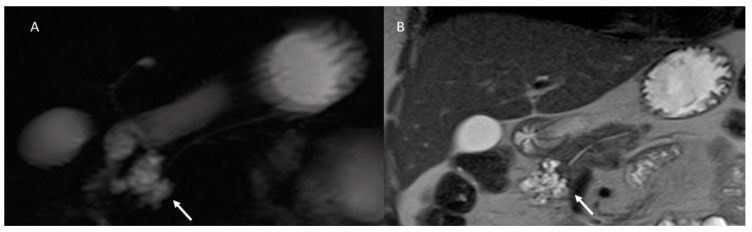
Abbreviated MRI assessment (MRCP: (A) and T2-W: (B) sequences) of pancreatic cistoadenoma, which is characterized by multicyclic lesions (arrow).
